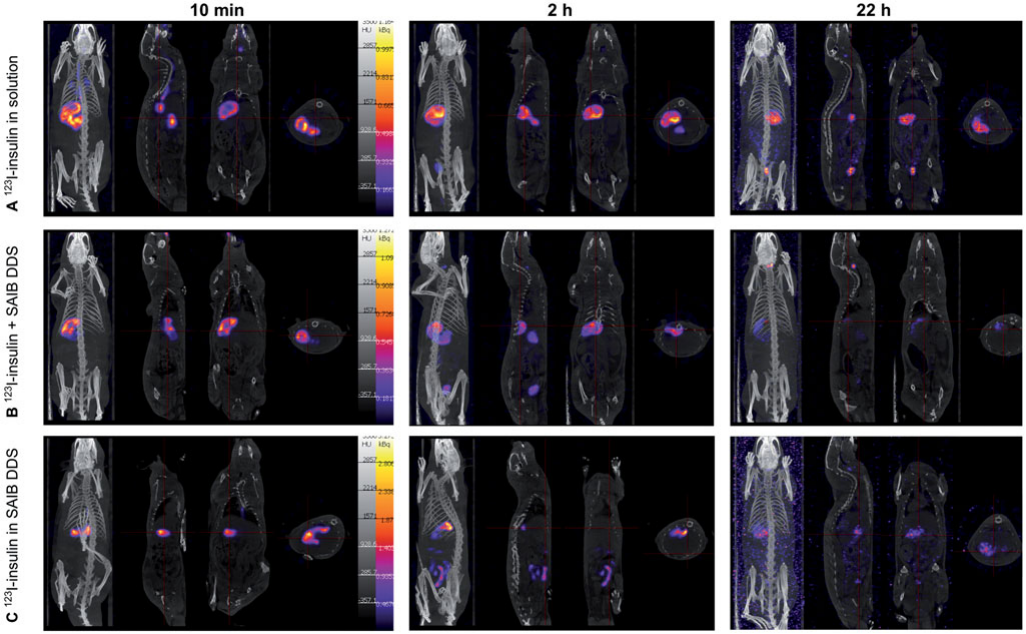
Representative SPECT/CT images showing the biodistribution of orally administered 123I-insulin 10 min, 2 h and 22 h after administration. The scale bar is the same for all images.
